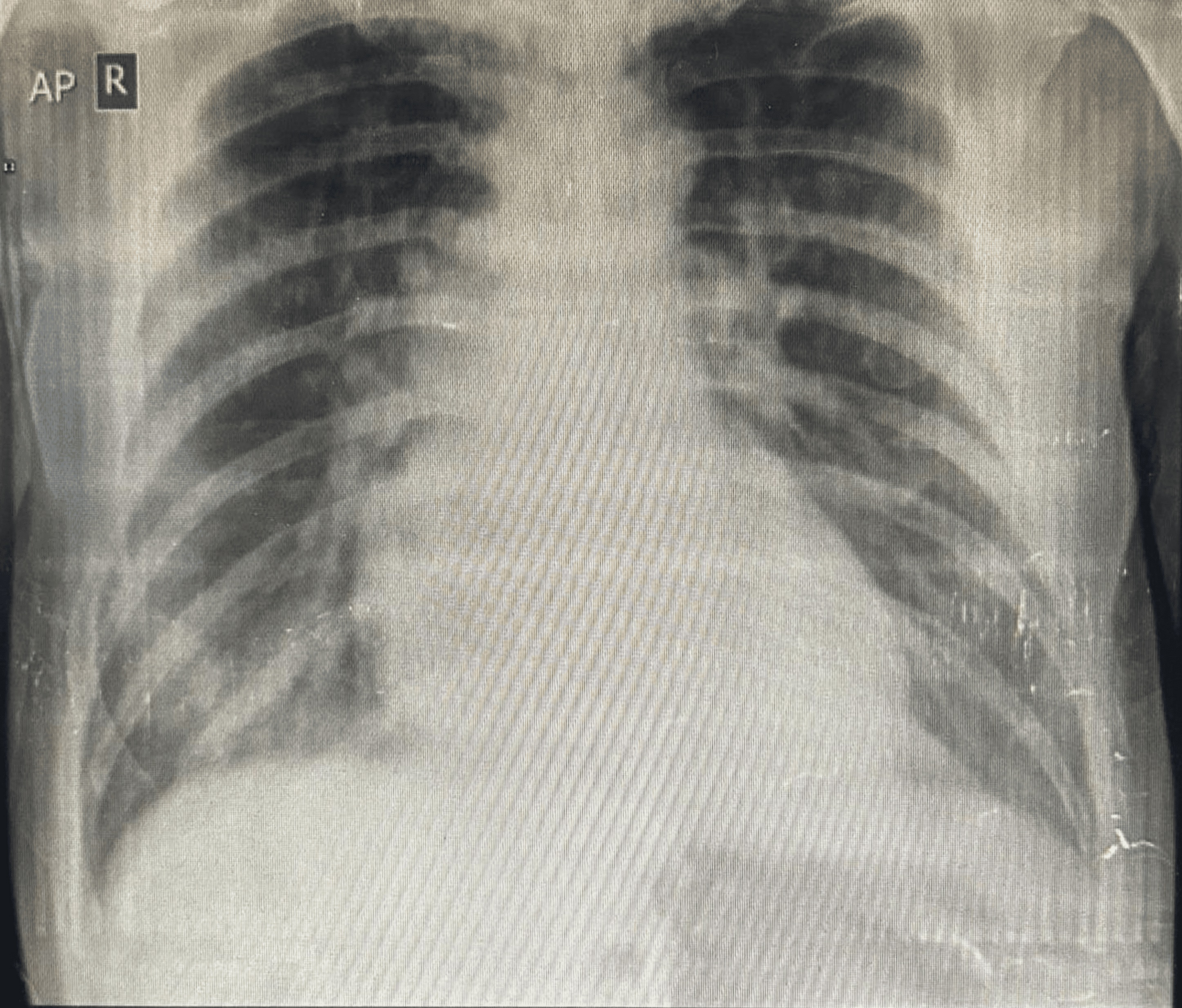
Portable chest X-ray at the second presentation in the ER of Al-Mahabba Hospital, showing cardiomegaly and bilateral hilar prominence suggesting pulmonary venous congestion.
